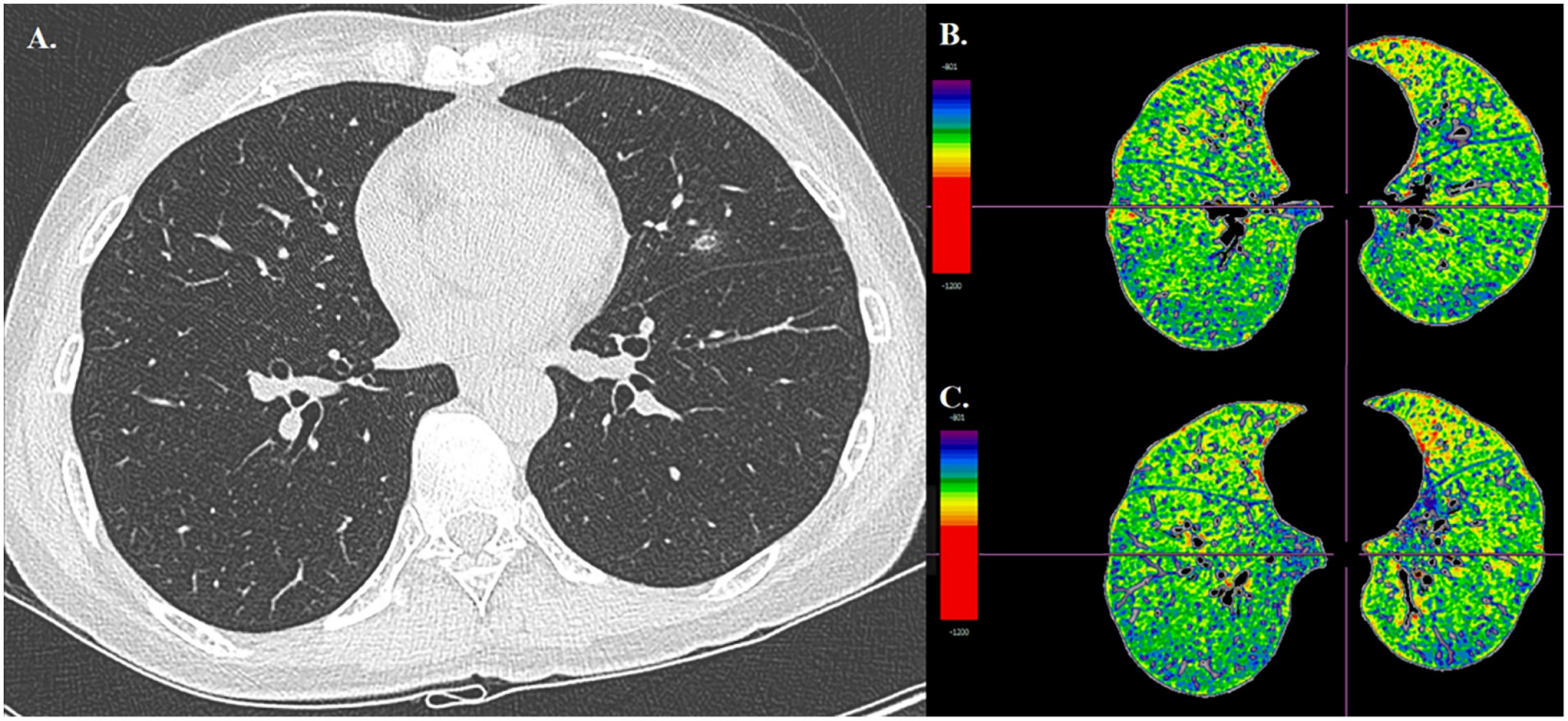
Representative case of prolonged air leak after anatomical segmentectomy. A 54 year-old female patient underwent anatomical segmentectomy for a persistent subsolid nodule approximately 1cm in left lingular segment (A). On pre-operative chest CT images, emphysema index of the entire lung is 11.6% and the lobe-specific emphysema index is 17.8% (B, C). Prolonged air leak occurred after lingular segmentectomy.
